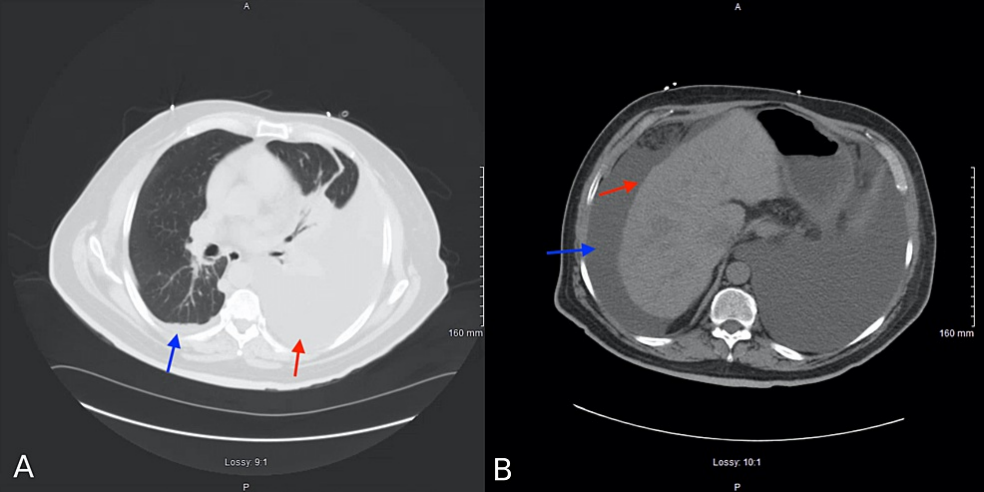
(a) Axial section of a computed tomography of the chest demonstrating a large left-sided pleural effusion with atelectasis (red arrow) and small right-sided pleural effusion (blue arrow). (b) Axial section of a computed tomography of the abdomen demonstrating nodular liver contour (red arrow) and large ascites (blue arrow).
